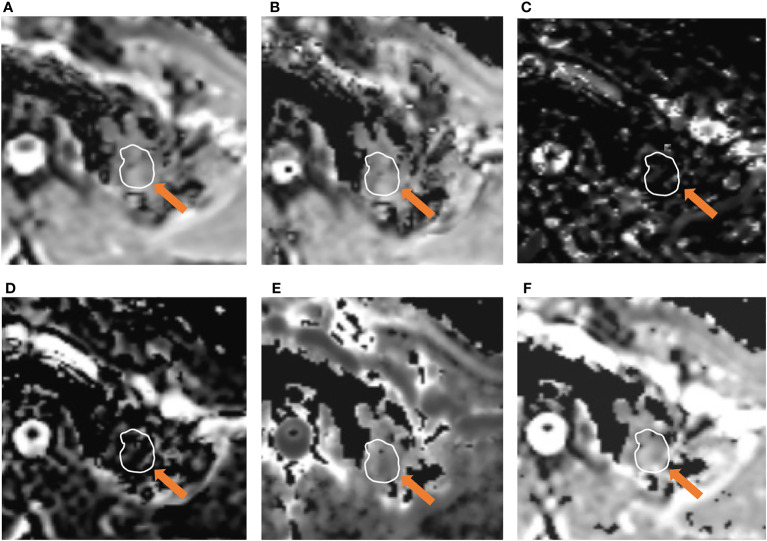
A 51-year-old male was diagnosed with pulmonary tuberculosis in the left upper lobe. Parameter map of ADC, D, D*, f, Kapp, and Dapp calculated from multiple b-value DWI data (A–F). The calculated mean values of ADC, D, D*, f, Kapp, and Dapp were 1.21×10^-3^ mm^2^/s, 1.13×10^-3^mm^2^/s, 8.74×10^-3^ mm^2^/s, 12.62%, 0.65, and 2.34×10^-3^ mm^2^/s, respectively.
